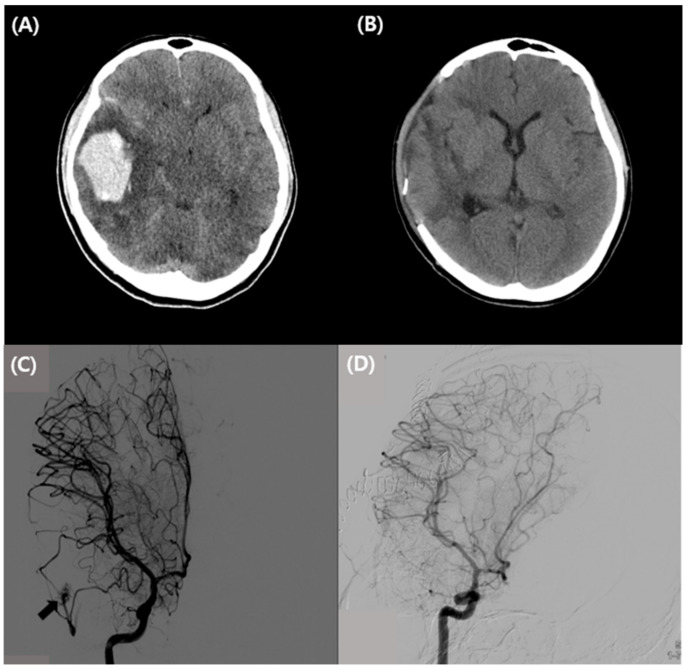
Initial and post-operative brain CT and TFCA images in the patient. (A) Preoperative axial image on non-enhanced brain CT, showing a 5.0 × 3.7 × 5.0 cm^3^-sized intracranial hemorrhage in the right temporal lobe with perilesional edema and mass effect with mild left side midline deviation. (B) Postoperative non-enhanced brain CT image 30 days after craniectomy showing marked resolution of a residual hematoma in the right cerebral hemisphere and improvements in perilesional edema and mass effect. (C) Preoperative TFCA showing that blood was supplied to the brain AVM by the right middle cerebral artery branch and drained into the right transverse sinus (arrow). (D) Post-operative TFCA 30 days after craniectomy showing removal of the AVM nidus and no residual shunt flow. Abbreviations: CT, computed tomography; TFCA, transfemoral cerebral angiography; and AVM, arteriovenous malformation.
